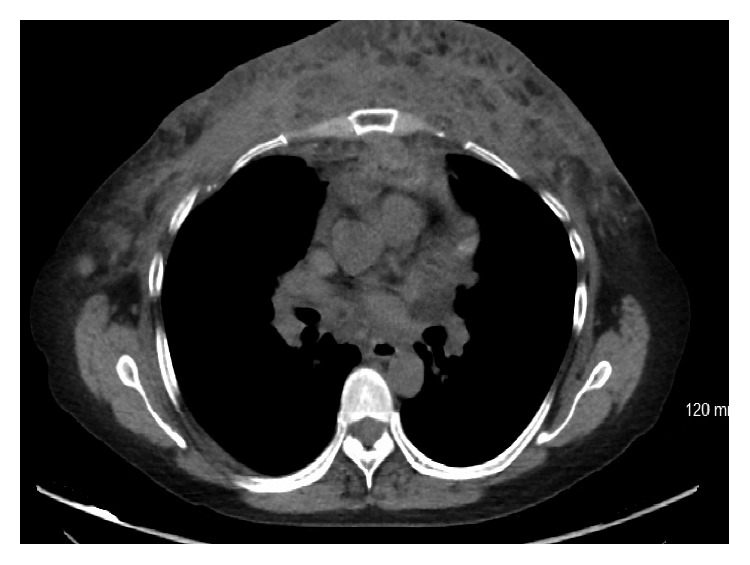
CT chest 2012: extensive Infiltration involving the mediastinum as far back as the spine, most pronounced anteriorly and extending throughout the chest to the diaphragm along with epicardial infiltration.
